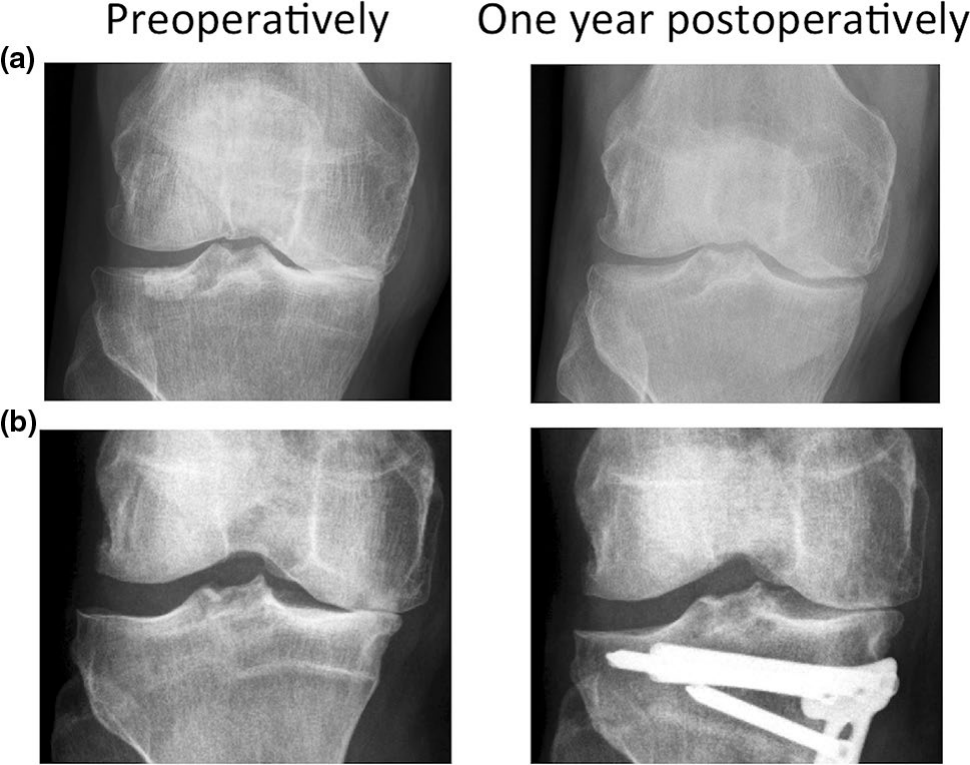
a Radiograph preoperatively (left) and 1 year post-operatively (right) of a representative patient treated with knee joint distraction. b Radiograph preoperatively (left) and 1 year post-operatively (right) of a representative patient treated with HTO
